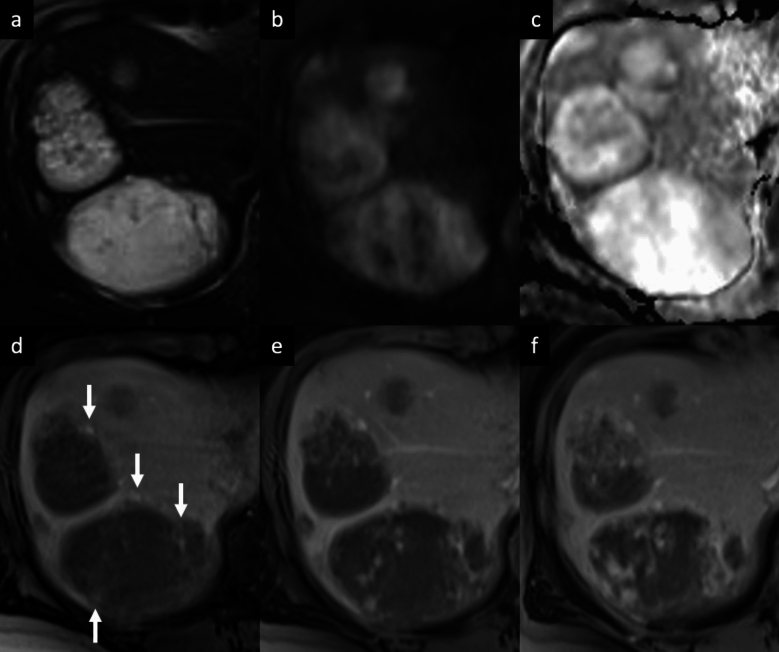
Hepatic angiosarcoma in a 35-years-old woman. a Axial fat-suppressed T2-weighted MRI shows multiple focal liver lesions in the right lobe with heterogeneous intermediate-to-high signal intensity. b On diffusion-weighted image the lesions demonstrate a heterogenous appearance, with mixed presence and absence of moderately hyperintense areas, displaying a heterogenous intralesional pattern on the apparent diffusion coefficient map (c). Extracellular contrast agent-enhanced MRI shows irregularly shaped and peripherally located foci of discontinuous enhancement on arterial phase (arrows, d), with an irregular flame-shaped both peripheral and central enhancement on portal venous phase (e) that progresses without a complete fill-in on delayed phase (f)
